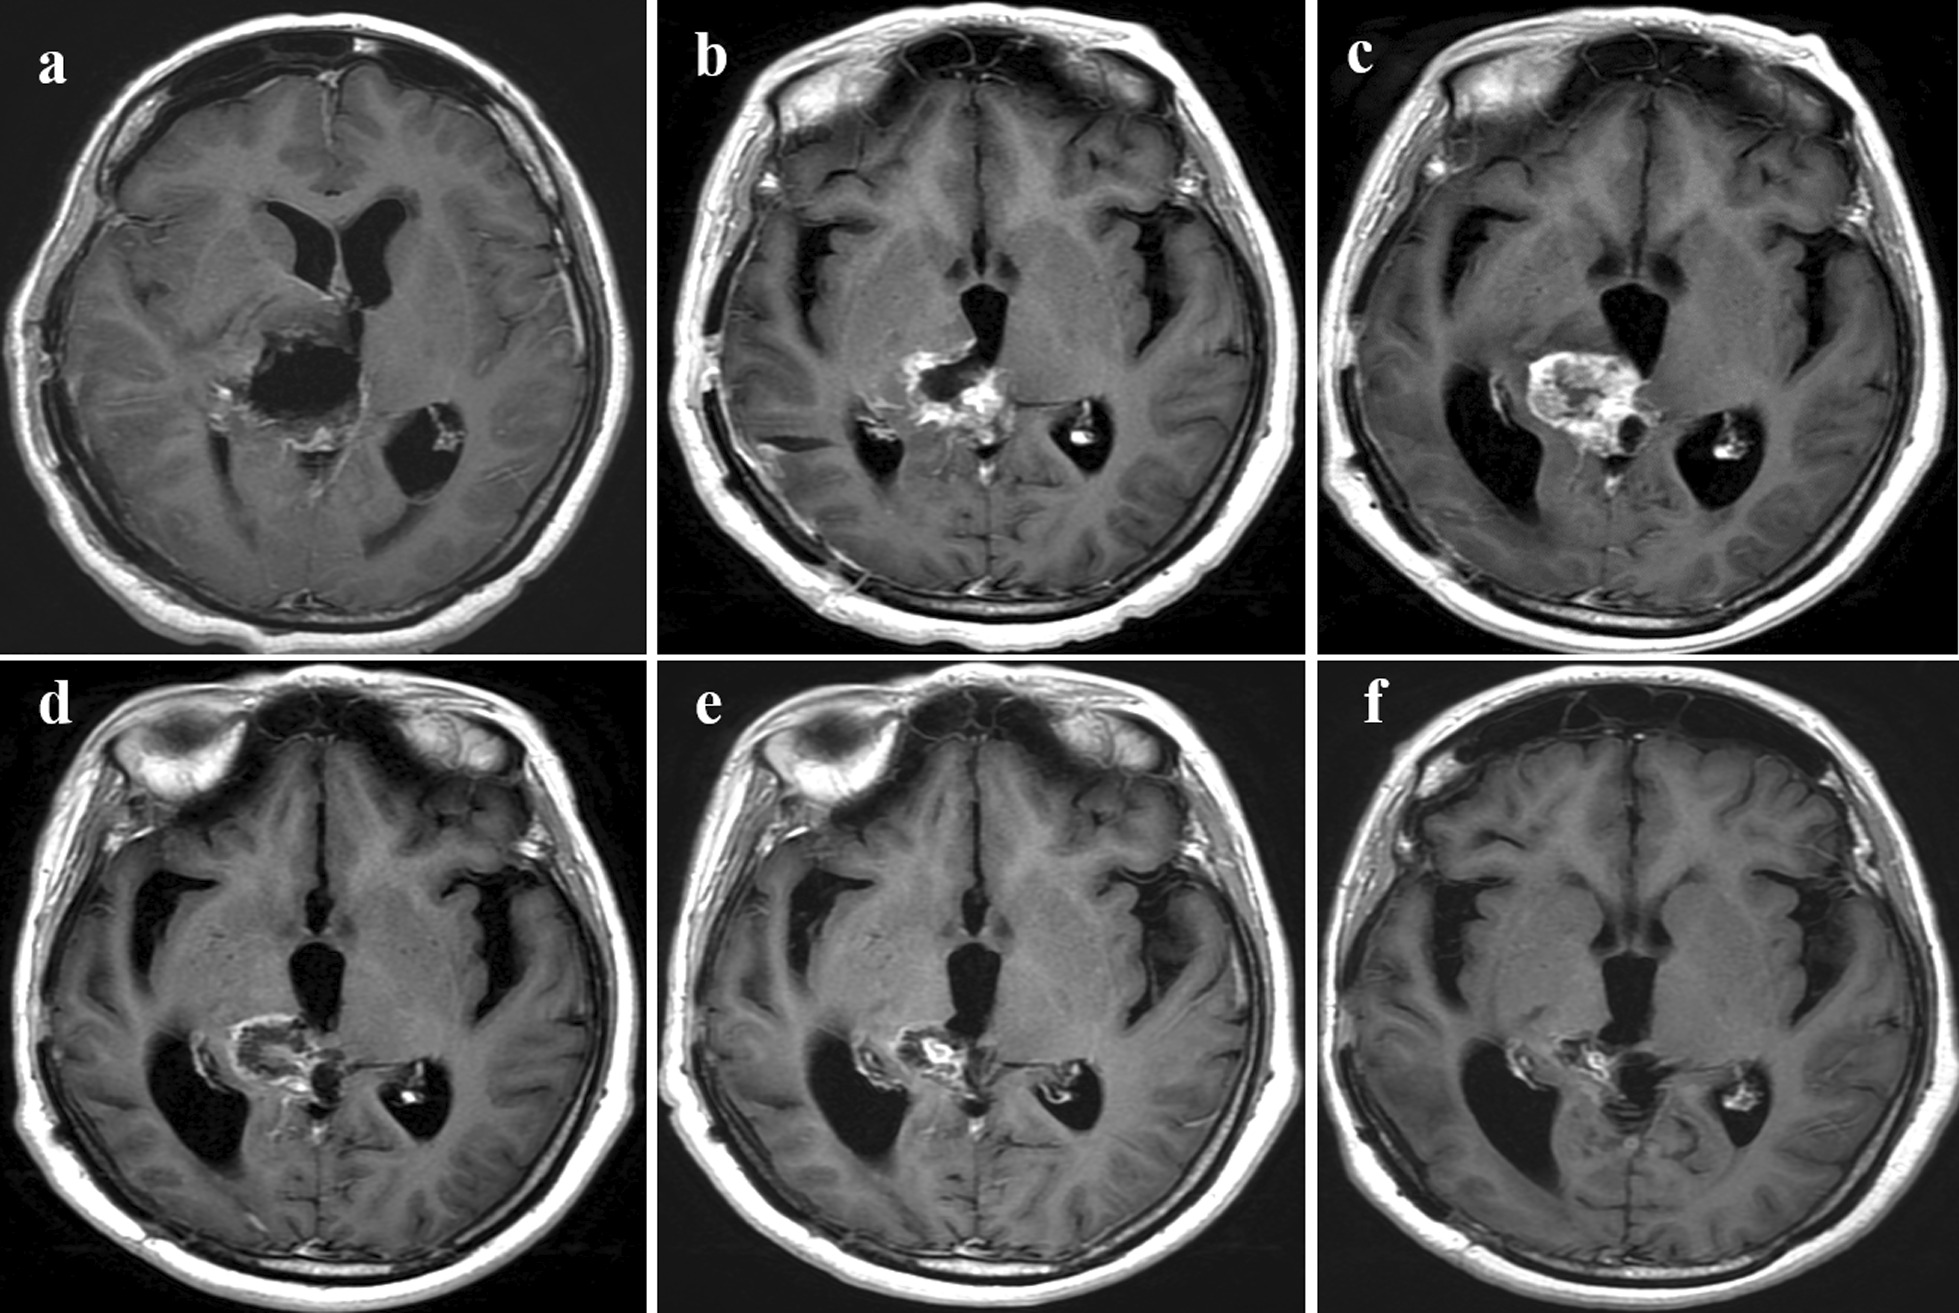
T_1_CE images showing pseudoprogression in a 45-year-old female patient with GBM. (a) Postoperative MRI (within 48 h after surgery) showing complete tumor resection. (b) Three days before CCRT, MRI showed mild enhancement of the cavity walls denoting surgical trauma-related changes. (c) Two months after CCRT, enhancement markedly increased. After CCRT and at the (d) 6-, (e) 8- and (f) 11-month follow-ups, the follow-up MR images demonstrated that the degree of lesion enhancement was reduced and the extent of enhancement was reduced. (CCRT: concurrent chemoradiotherapy)
